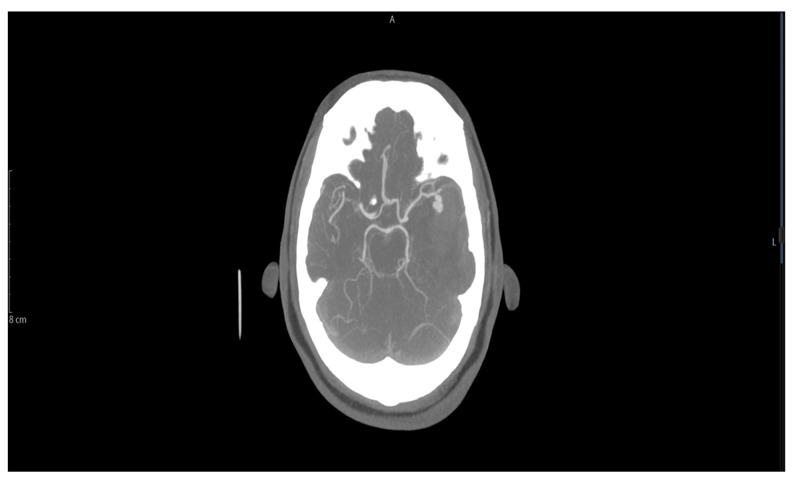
CT angiography of the head and neck showed a 10.7 x 9.6 x 7.6 mm bilobed-shaped fusiform aneurysm arising from the distal aspect of the posterior trunk of the left middle cerebral artery CT: computed tomography
